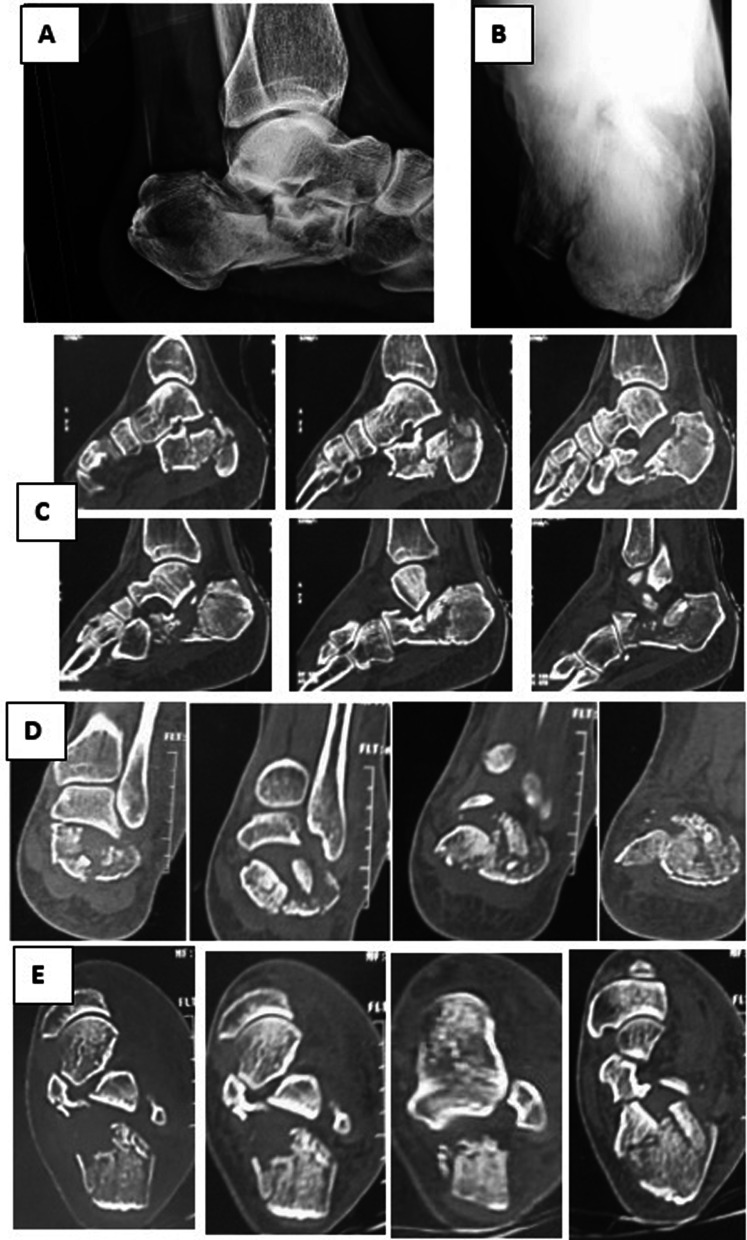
Preoperative radiographs of a displaced intra-articular calcaneal fracture: A lateral plain ankle X-ray showing the depressed articular surface of the calcaneus; B axial view of the calcaneus; C, D, E sagittal, coronal and axial CT cuts, respectively
